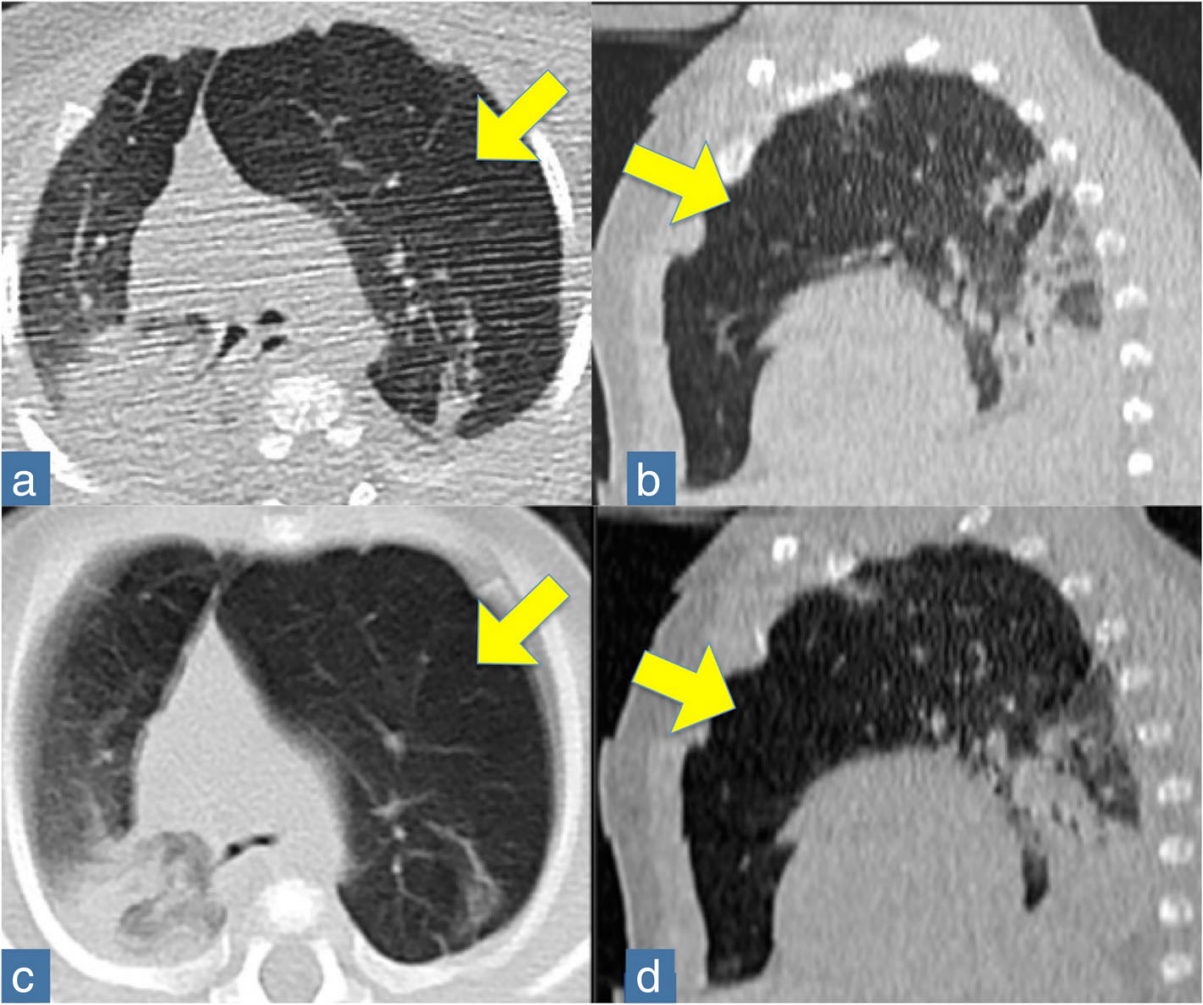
CT thorax at admission (Panels a, b) and two months later (Panels c, d). The arrows indicate the hyperinflation area. Panels a, c: axial position; Panel b, d: sagittal position
